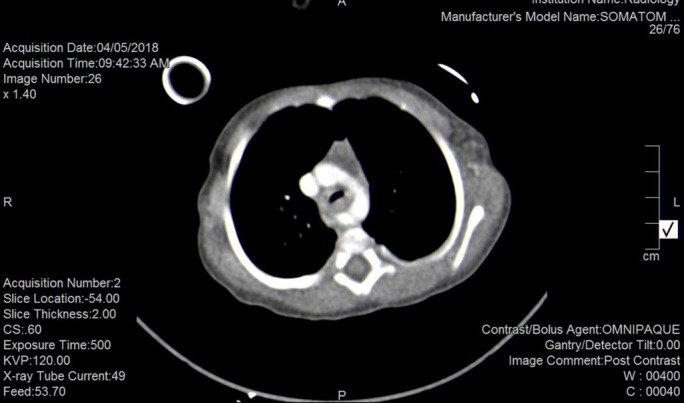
Axial computed tomography chest image showing a complete aortic sling, which appears to encase the trachea and oesophagus.
